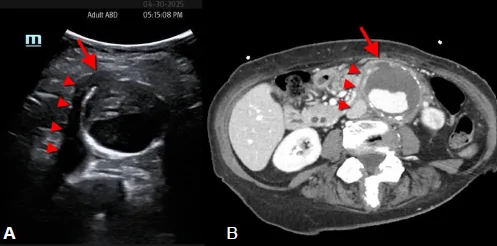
Point-of-care ultrasound and computed tomography angiography (A) Transabdominal longitudinal ultrasound view of the abdominal aorta showing a markedly dilated aneurysm with focal wall discontinuity (arrow) and adjacent hypoechoic collection (arrowheads). (B) Computed tomography angiography showing focal aortic wall disruption (arrow) with an adjacent retroperitoneal hematoma (arrowheads).
